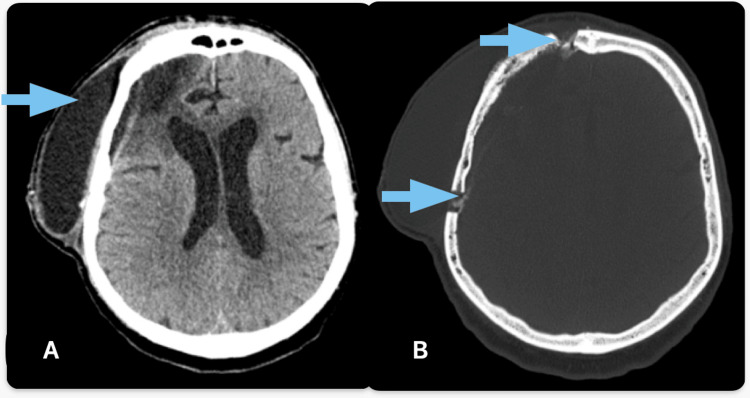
Postoperative dehiscence lesion Tissular window (A) of CT scan highlights the postoperative dehiscence lesion (blue arrow) at the level of pterional flap (B, blue arrows indicate trephine holes), shown on bone window
